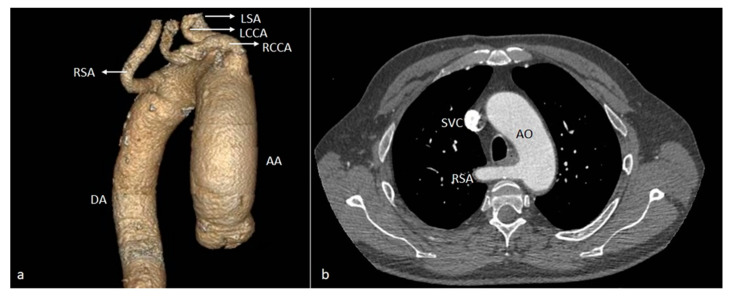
VR (a) and axial (b) CT images showing the aberrant origin of the right subclavian artery from the medial wall of the aortic arch with a retroesophageal course. AA—ascending aorta. DA—descending aorta. RCCA—right common carotid artery. LCCA—left common carotid artery. RSA—right subclavian artery. LSA—left subclavian artery. SVC—superior vena cava. AO—aorta.
